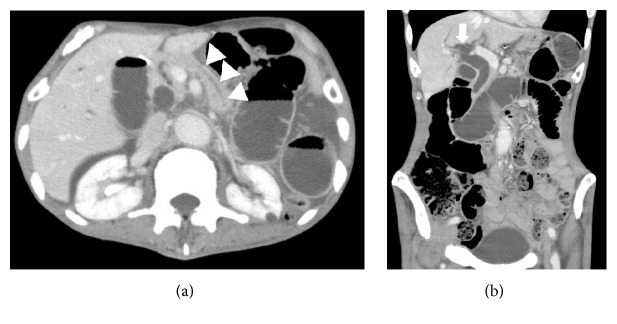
Computed tomography scan images with axial and coronal views showing slight dilation of the main pancreatic duct (arrow heads) and the intrahepatic bile duct (arrow).
